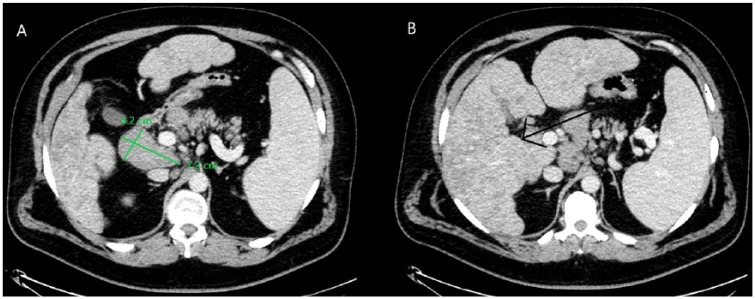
(A) Computed tomography (CT) scan of abdomen and pelvis with intravenous contrast enlarged precaval lymph node measuring 7.2 × 4.2 cm. (B) CT of abdomen and pelvis with intravenous contrast revealing diffuse nodular hepatomegaly and splenomegaly.
